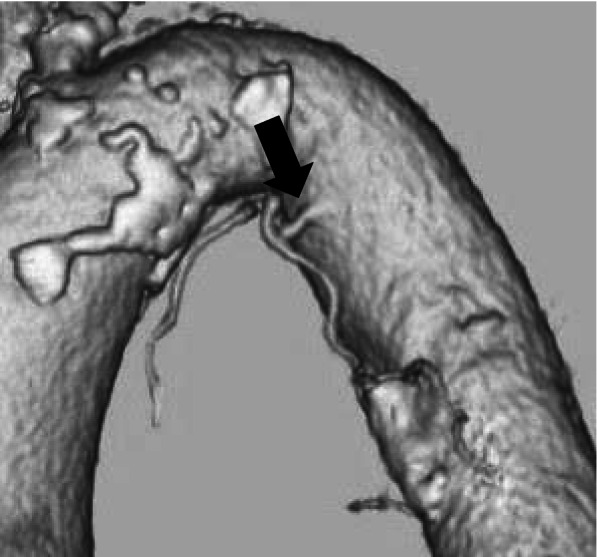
Computed tomography angiography of bronchial arteries. The arrow indicates the common trunk of bronchial arteries which arose from the thoracic aorta
